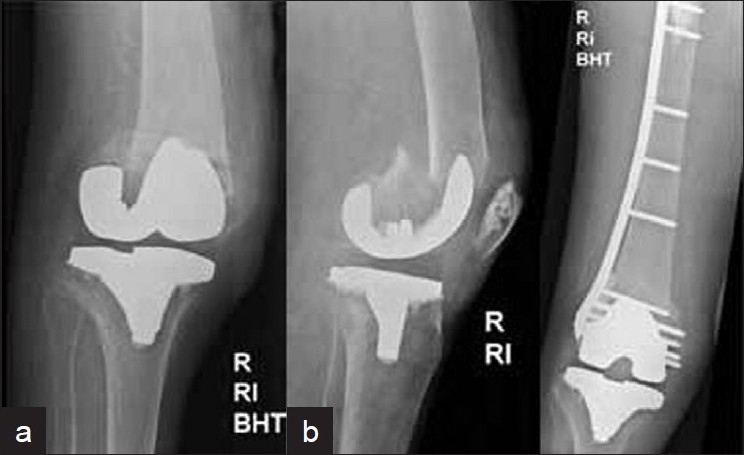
(a) Anteroposterior and lateral radiographs of right knee in a 88-year-old female with total hip and knee arthroplasty who fell and suffered a periprosthetic distal femur fracture. (b) Open reduction and internal fixation using LISS plate osteosynthesis was done and uneventful osseous healing as depicted was obtained
